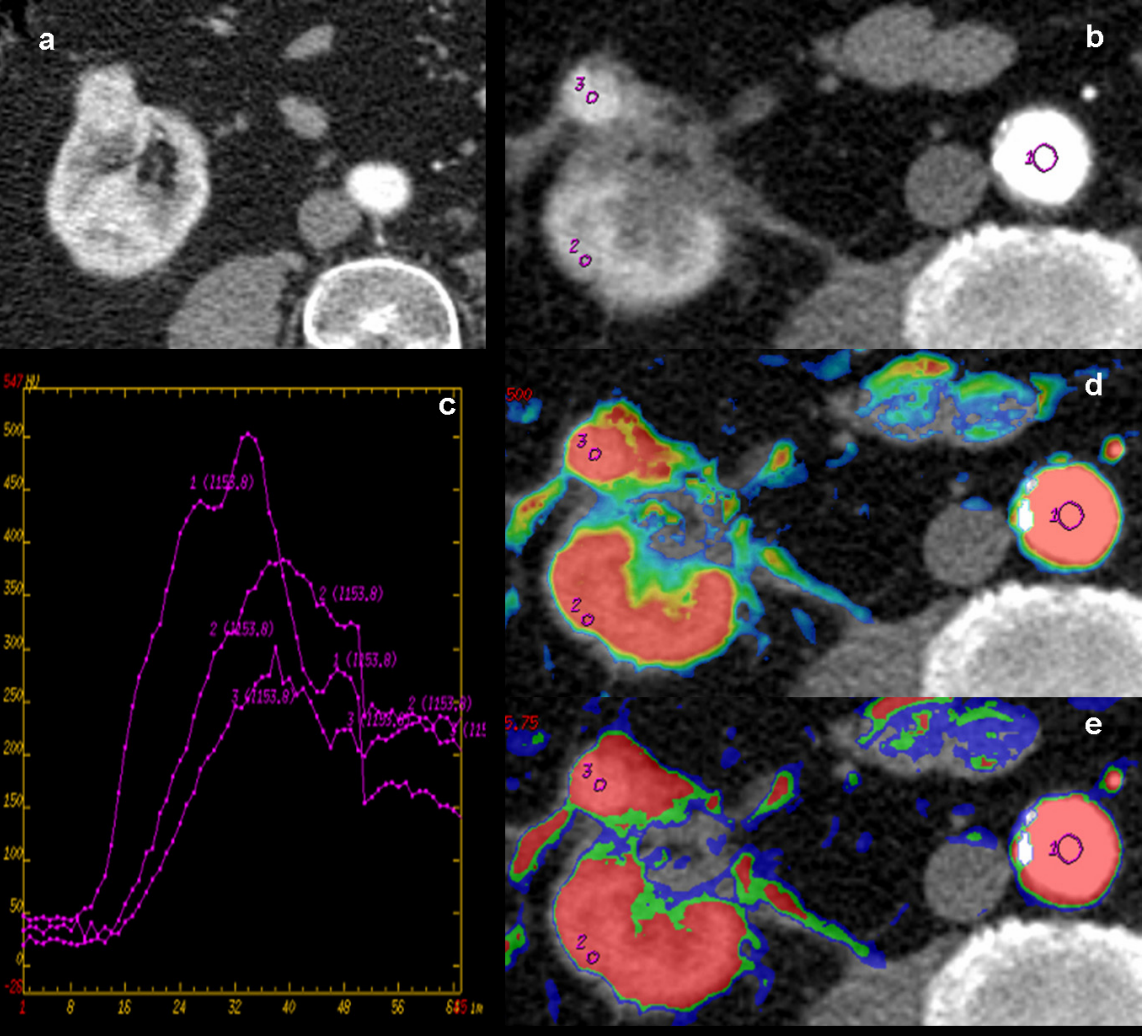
Residual renal cancer cell (RCC) in right kidney, six months after cryoablation. Pre-treatment contrast-enhanced cortico-medullary phase CT scan (a) shows exophytic solid tumor with heterogeneous contrast-enhancement. Post-treatment perfusional CT (b) shows a nodular enhancing component (ROI 3) in the medial portion of the ablation zone with peripheral linear enhancement in the peri-renal fat, suggestive for residual tumour. Regions of interest were placed in abdominal aorta (ROI 1), normal renal cortex (ROI 2) and nodular solid enhancement area (ROI 3) to measure perfusional parameters. Time/Density curve (c) shows a typical contrast enhancement pattern in residual tumour area with fast and early wash-in, a plateau trend and a slow, progressive and uniform wash-out (curve 3). Color maps superimposed on gray-scale images (d, Blood Volume, BV; e, Blood Flow, BF) of right kidney show high colour encoding in corresponding residual tumour area: (d) BV (mean, 140,68 ± 24,48 mL/100 g wet tissue/min), (e) BF (mean, 562,72 ± 97,96 mL/100 g wet tissue).
